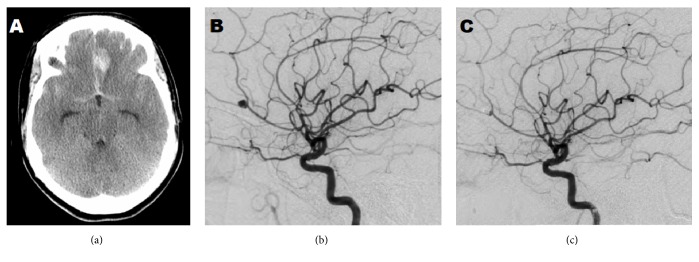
Imaging study upon patient's arrival at the hospital in 2012. (a) CT head without contrast showing diffuse subarachnoid hemorrhage that originated from an aneurysm identified by (b) Cerebral angiogram. (c) Cerebral angiogram imaging after coiling of an aneurysm.
